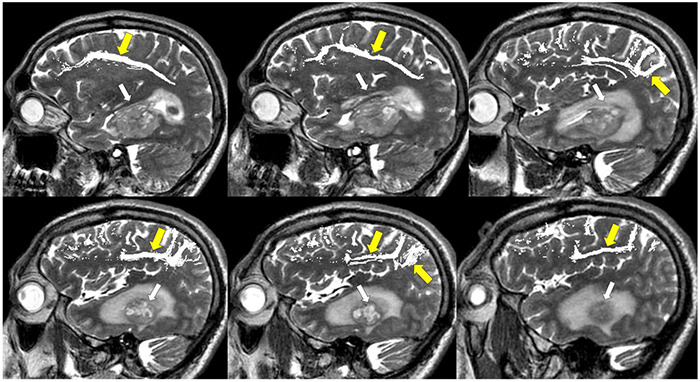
Tractography: superior longitudinal fasciculus. Sagittal T2-weighted images show glioblastoma centered in the hippocampus and parahippocampal gyrus (white arrows). Overlay of tractography of superior longitudinal fasciculus (yellow arrows) demonstrates sparing of the tract by the tumor.
